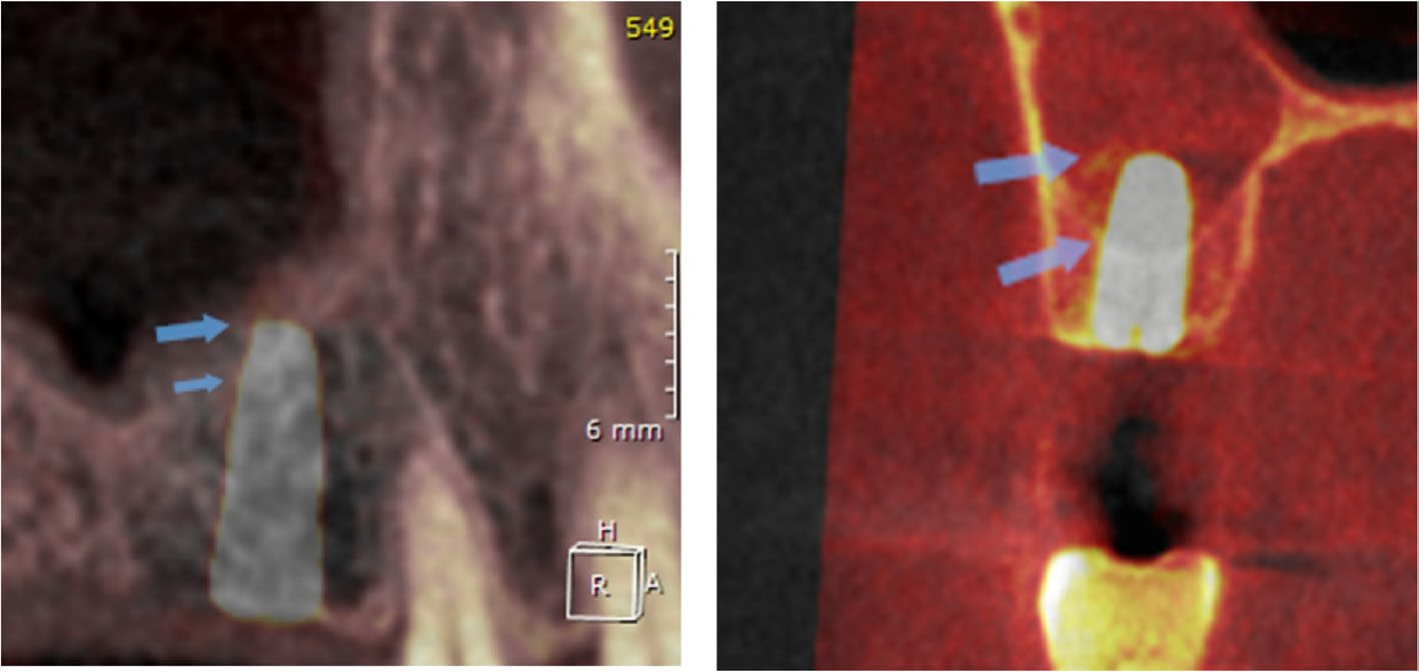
Superimposition of Pre&post-operative CBCT to compare vertical bone gain. Per & post-operative Sinus floor level is marked by the arrows
